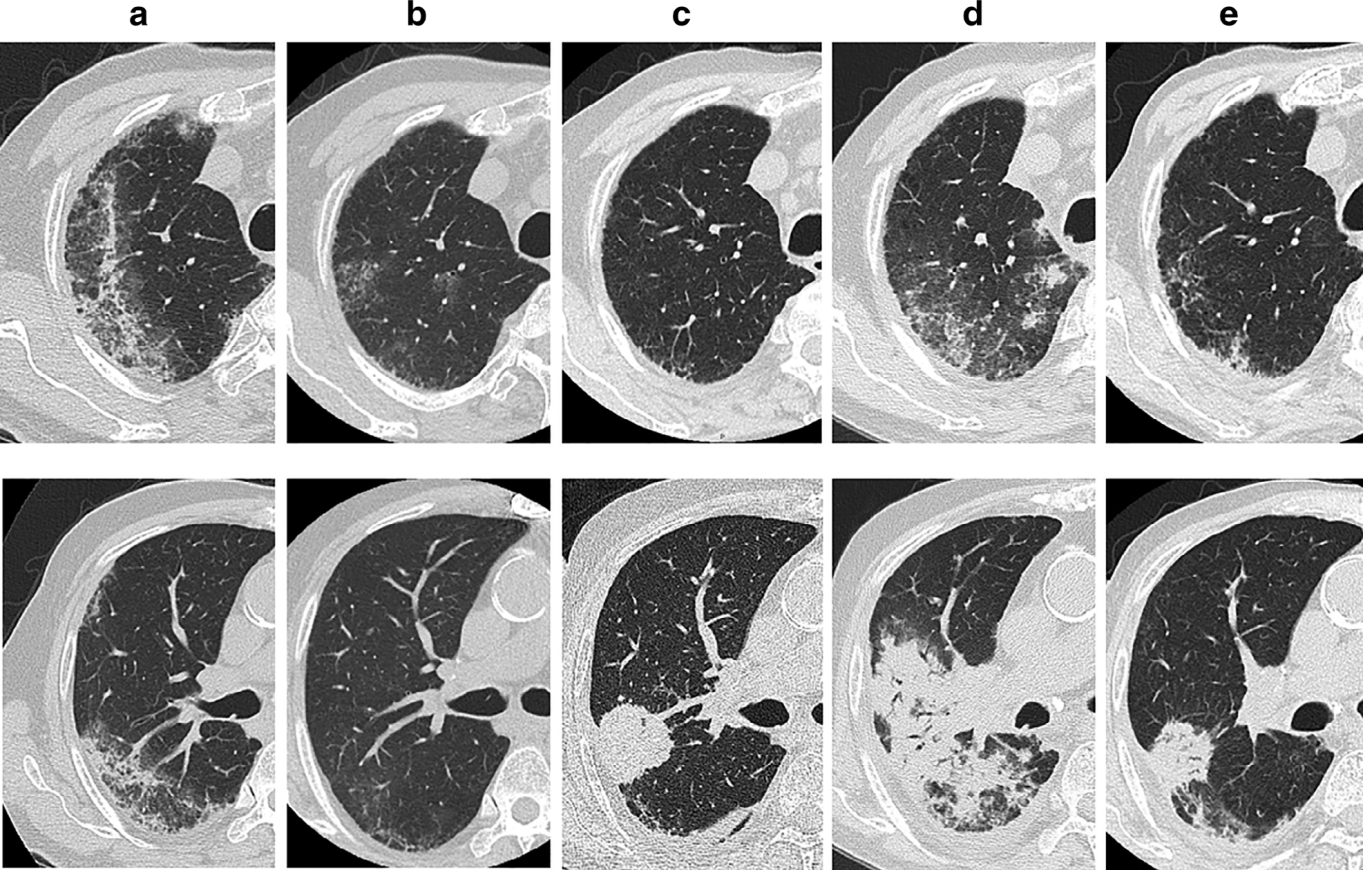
High resolution computed tomography (CT) of the chest at (a) organizing pneumonia (OP) occurrence, (b) after OP amelioration, (c) diagnosis of lung cancer, (d) relapse of OP, and (e) after amelioration of OP relapse
